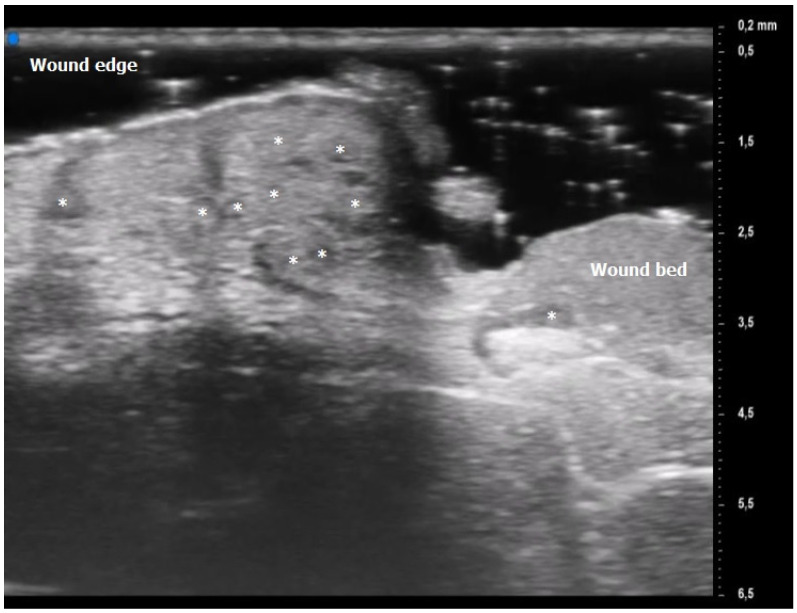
Pyoderma Gangrenosum (PG) Ultra-High Frequency UltraSound (UHFUS) features during the inflammatory phase (T0): oval hyperechoic structures (*) surrounded by hypoechoic borders predominantly located at the ulcer edge.
